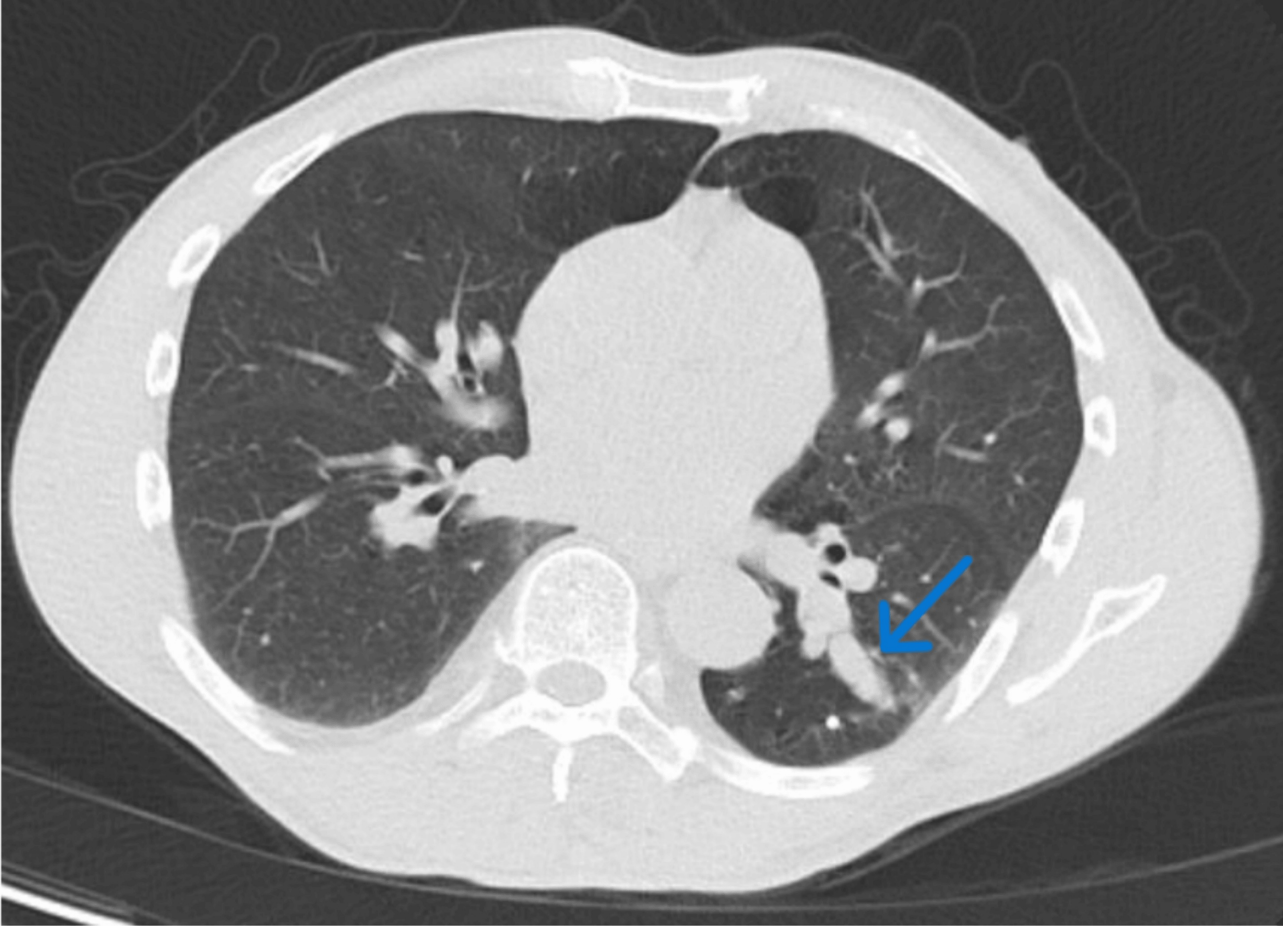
CT chest demonstrating scar-like, mucus-impaction appearance (blue arrow) of left lower lobe lesion.
